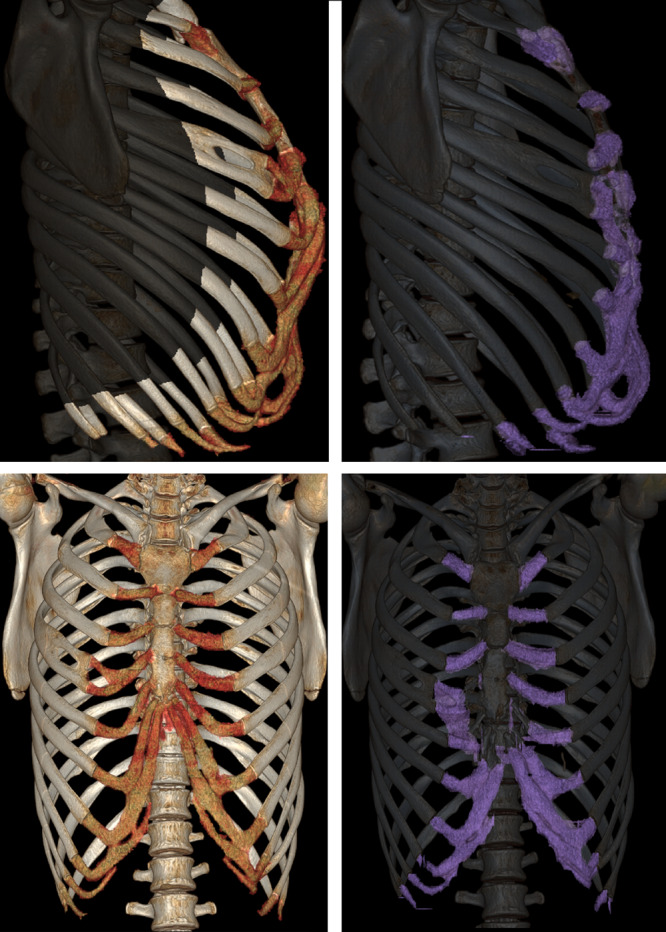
Three-dimensional volume-rendered computed tomography thorax imaging of the same patient as in Fig. 1, presurgery (left) and at 6 months post-surgery (right).
